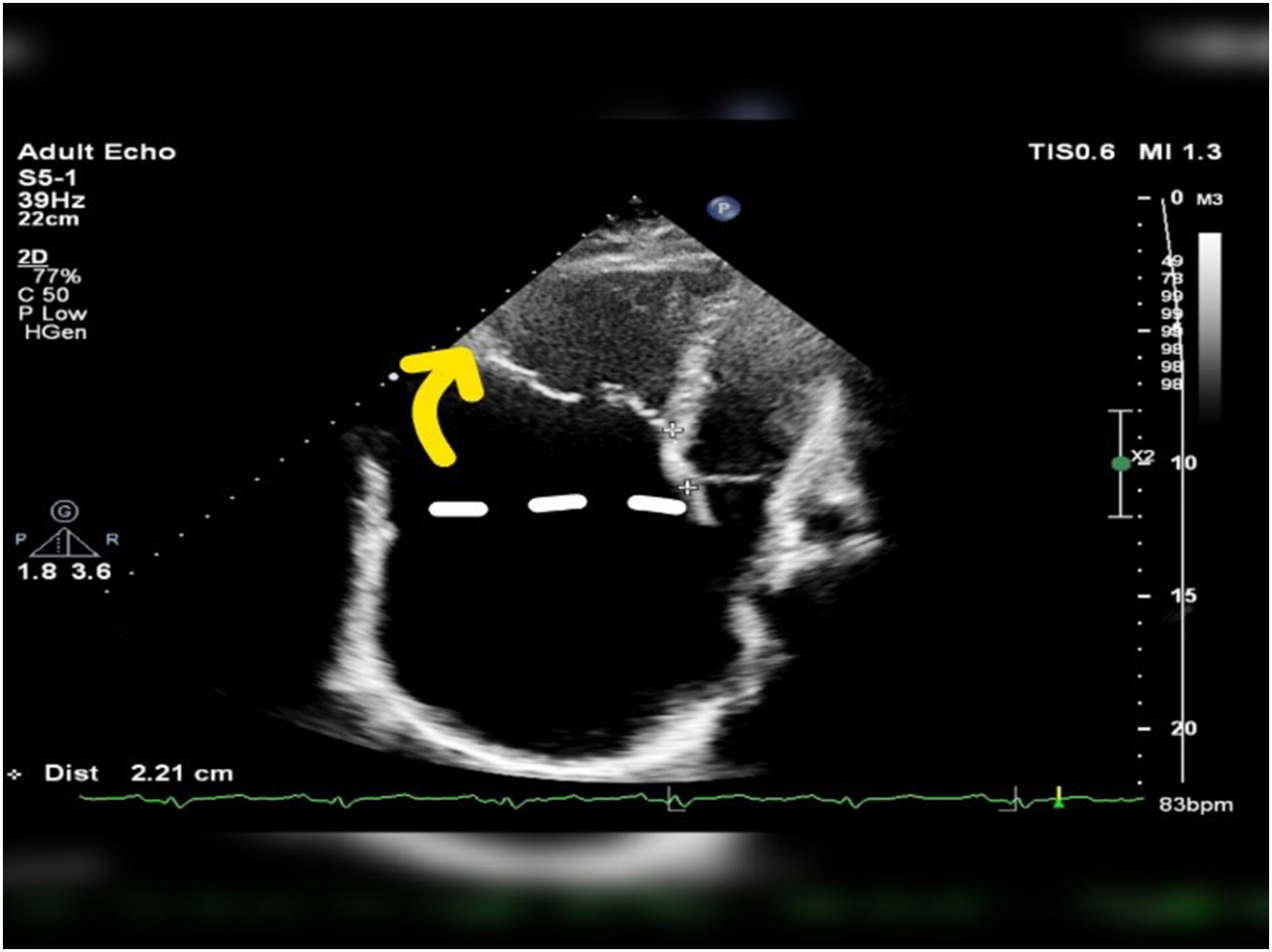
Apical 4chamber view showing displacement of anterior tricuspid leaflet (5.3 cm, yellow arrow) relative to tricuspid annulus in addition to displacement of septal leaflet (2.21 cm).
